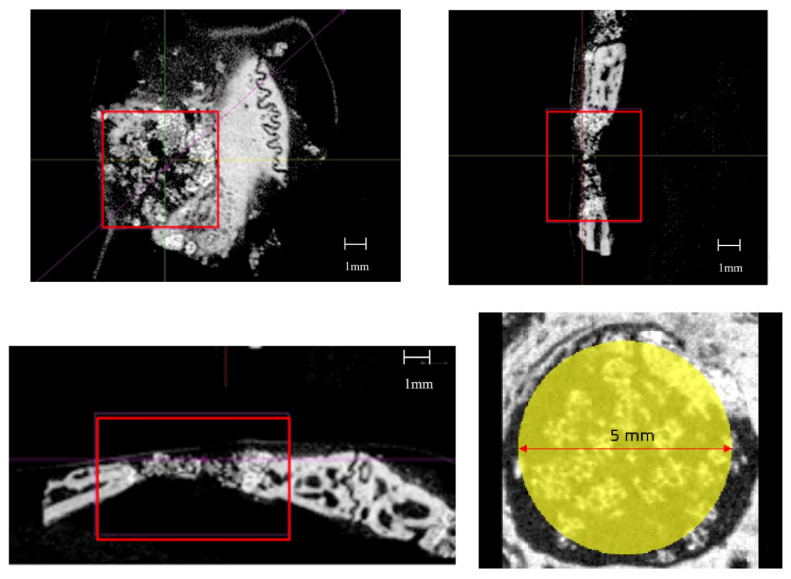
Micro–CT images and ROI.
